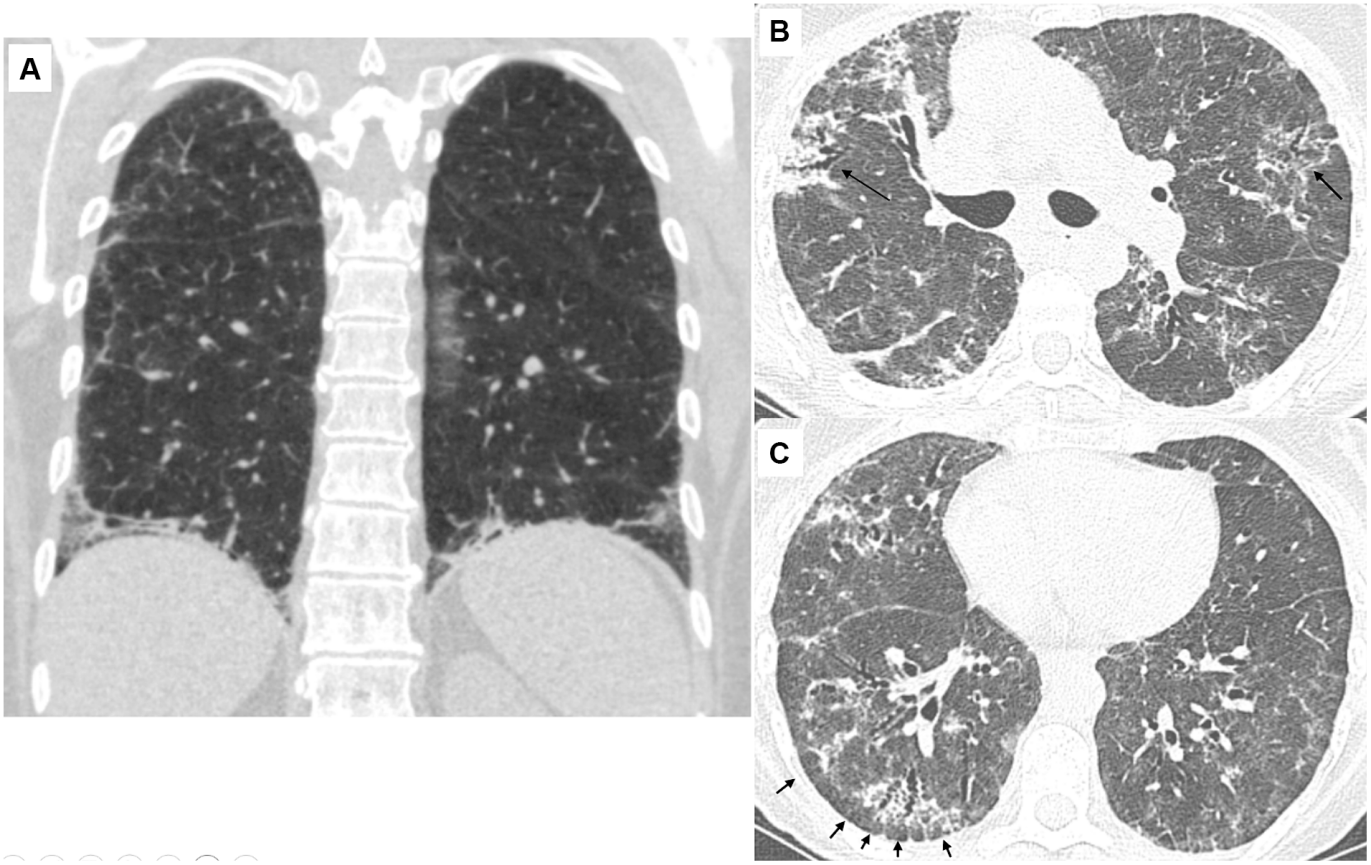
Characteristic CT findings of the lungs in patients with antisynthetase syndrome. (A) 56-year-old female (anti-Jo-1 positive). Coronal view. There is reticulation with ground-glass opacities and traction bronchiectasis. Note the extreme bibasilar location of the abnormal findings that seem to “hug” or “pancake” the diaphragms. This finding is considered highly suggestive of underlying polymyositis/dermatomyositis, especially antisynthetase syndrome. (B) 66-year-old female (anti-PL-7 positive). There are diffuse ground-glass opacities resulting in a mosaic pattern. There are also consolidative areas centered around bronchi (arrows) that have the characteristic corkscrew appearance in line with traction bronchiectasis. The presence of mosaic attenuation and bronchocentricity can erroneously support a diagnosis of hypersensitivity pneumonitis, especially in patients with a history of exposure to an inciting antigen. (C) 66-year-old female (anti-PL-7 positive). There are diffuse ground-glass opacities and traction bronchiectasis with the characteristic cork-screw appearance. Note that the fibrotic changes are located in the periphery of the lungs with relative sparing of the immediate subpleural parenchyma. The subpleural sparing is subtle in the left lung but evident in the right lung (arrows).
